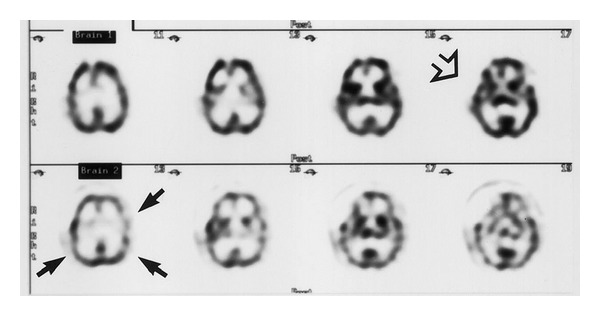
SPECT. Initial transaxial SPECT images (row 1) showing only a postoperative defect from clipping of an ACA aneurysm, demonstrated by the open arrow. Follow-up transaxial SPECT images (row 2) show diffuse hypoperfusion, indicating moderate vasospasm, demonstrated by the closed arrows. The figure is borrowed from [56].
